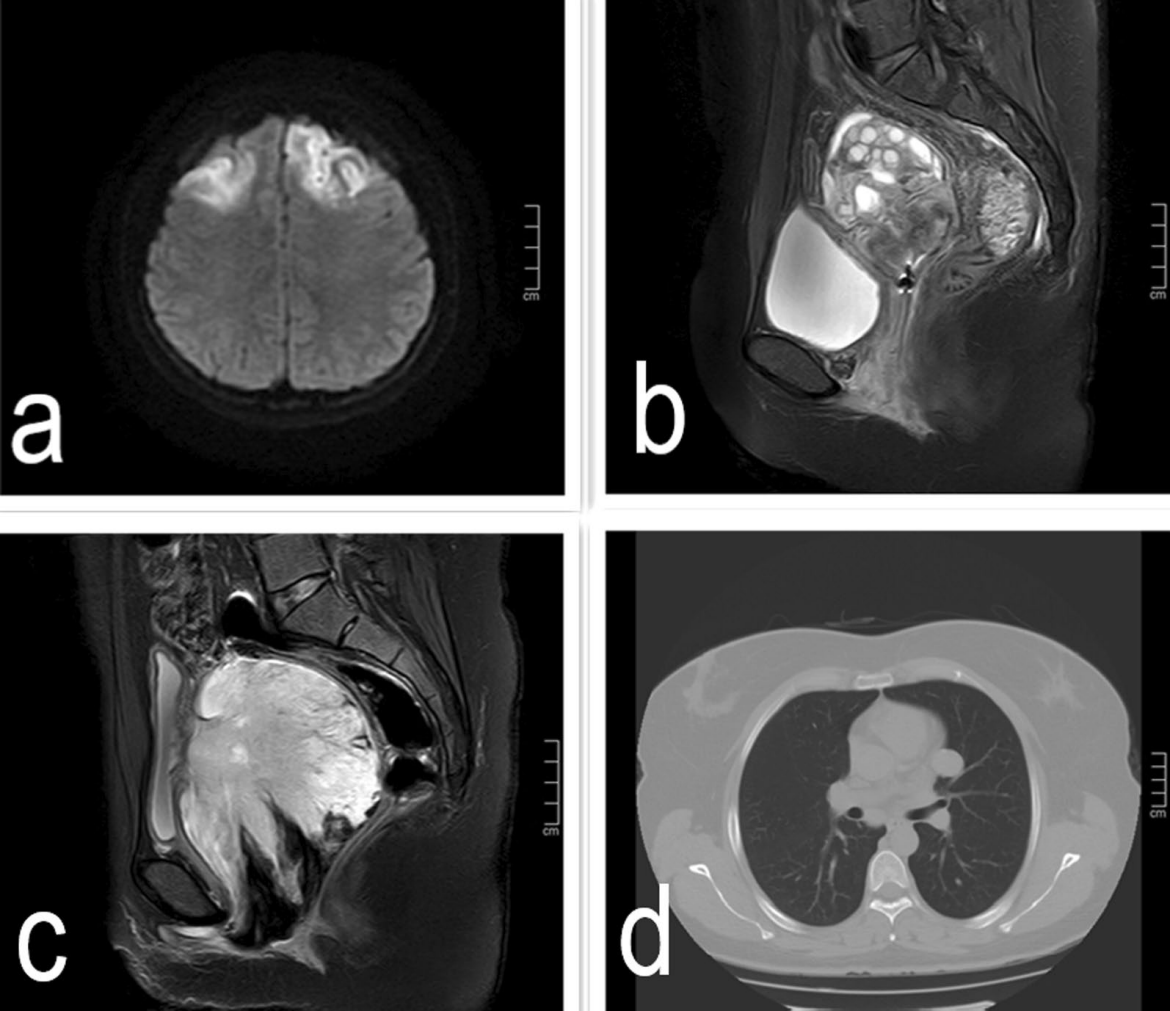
a Head CT scan: venous sinus thrombosis at admission; b pelvic MRI scan: pelvic recurrence two and a half months post hysterectomy: recurrent tumour; c pelvic MRI scan: pelvic recurrence five months post hysterectomy; d lung CT scan: lung metastasis five months post hysterectomy
